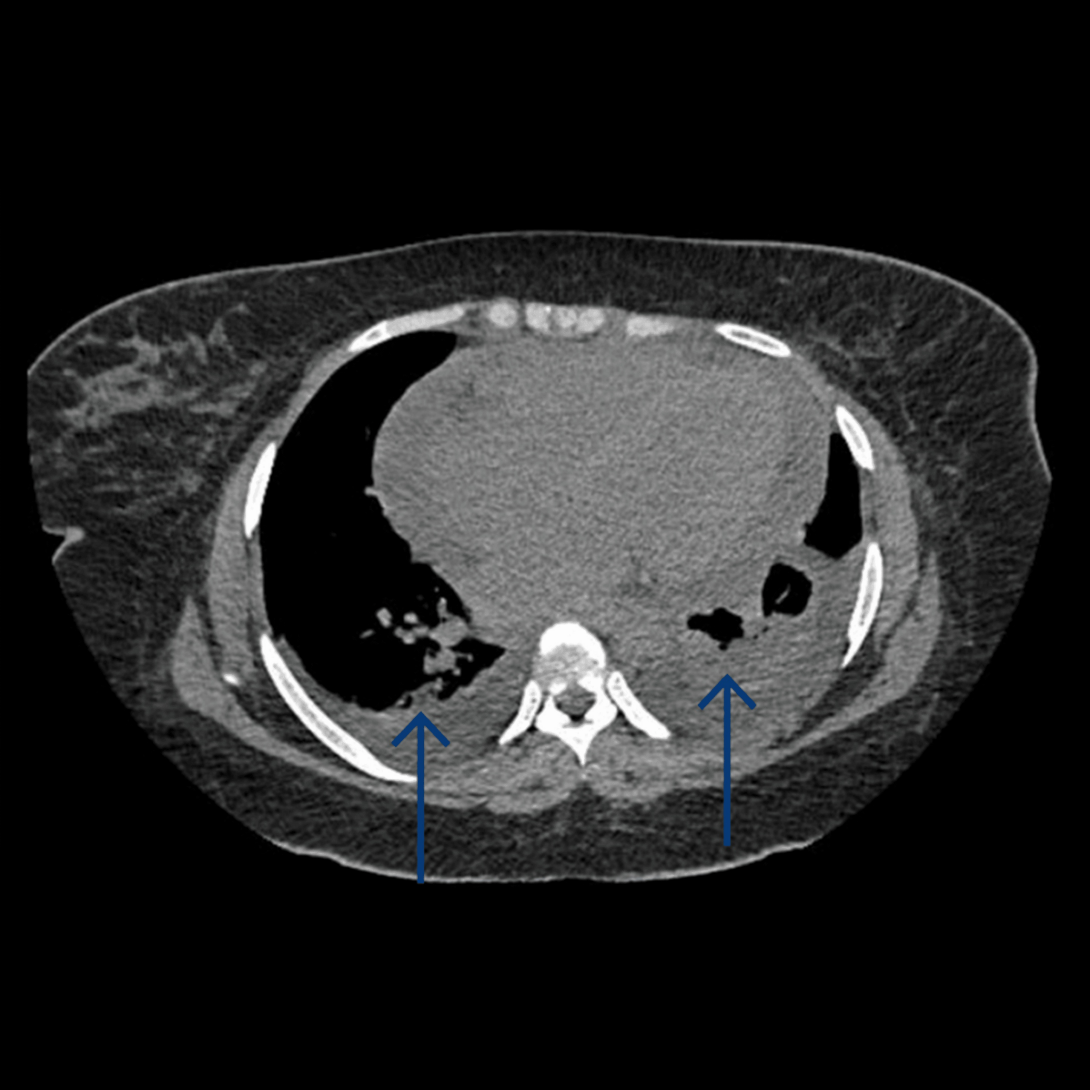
CT of the chest showing cardiomegaly and pulmonary edema.
